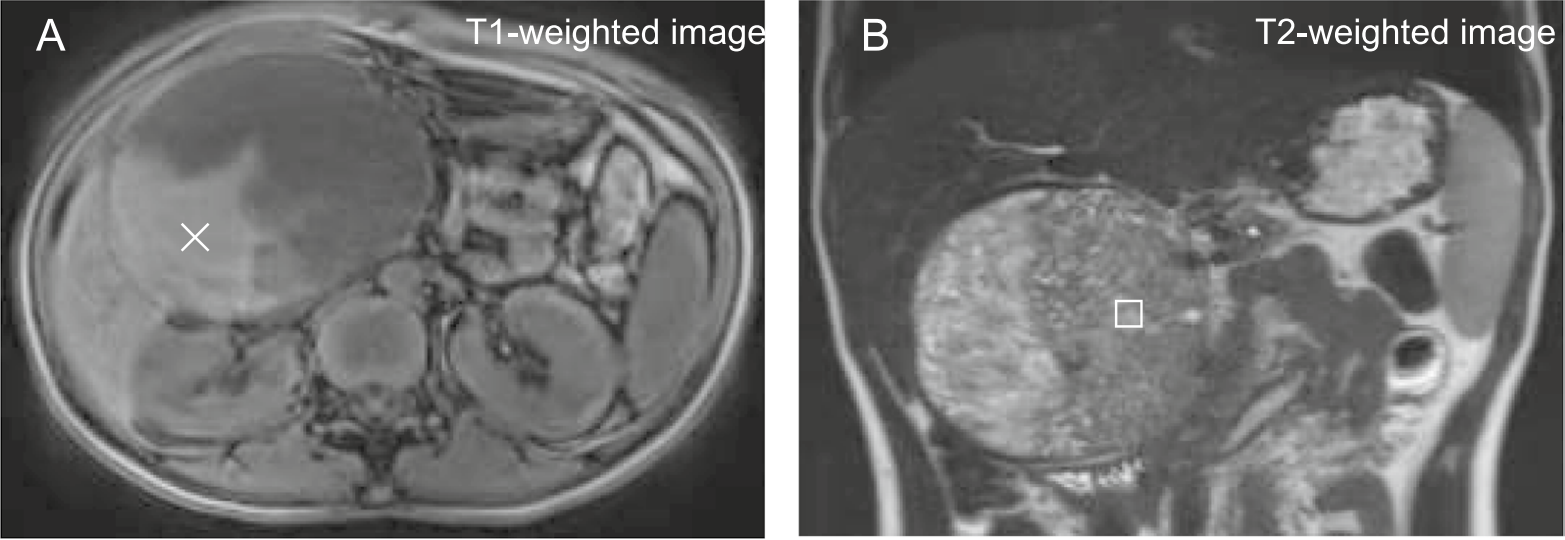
MRI on admission. The tumor’s internal structure is heterogeneous, and the T1-weighted image (A) shows a high signal (×), suggesting hemorrhagic changes. In the enhanced area, the T2-weighted image (B) shows predominantly intermediate signals (□), with a mixture of vesicular and slit-like high-signal areas. Therefore, the findings show a mixture of a substantial mass and hemorrhagic and degenerative necrotic areas. MRI, magnetic resonance imaging
